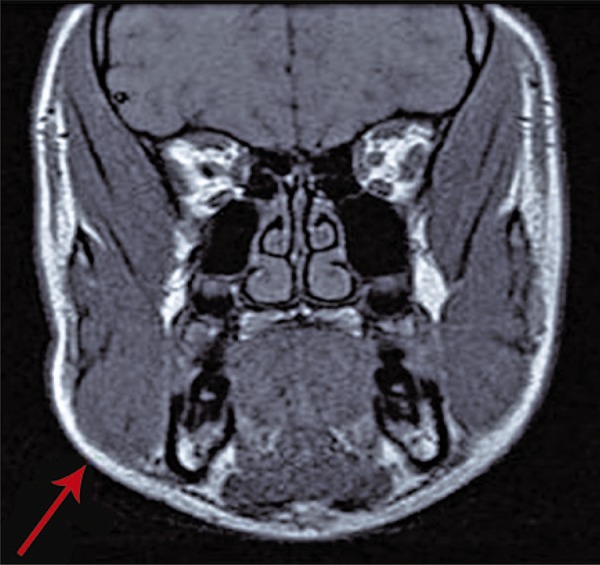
Coronal magnetic resonance imaging showing asymmetry of the right masseter muscle
